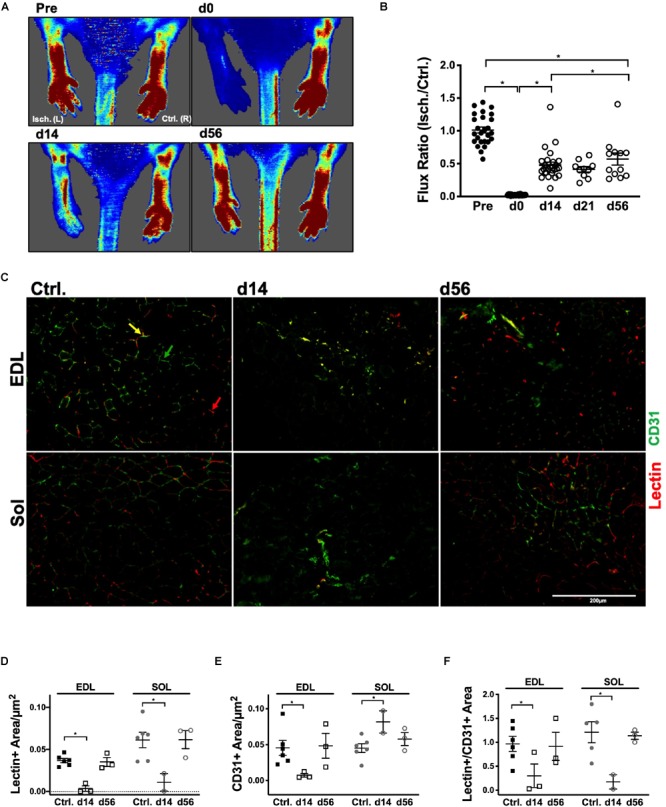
Restoration of peripheral blood flow and muscle capillary perfusion. (A) Representative laser Doppler perfusion images (LDPI) of the ischemic left [Isch. (L)] and control right [Ctrl. (R)] plantar paws in the prone position at baseline, d0, d14, and d56. (B) Quantification of flux measurements at baseline, d0, d14, d21, and d56 represented as a ratio of the left (Isch.) over right (Ctrl.) limbs. (C) Representative 20× immunofluorescent images of systemically DyLight 594 conjugated GS-IB_4_ lectin, which indicates vessels which were actively perfused at the time of sacrifice (red), and cluster of differentiation 31 (CD31^+^, PECAM-1; green), which indicates total endothelial cells, in transverse sections of the control, d14 and d56 Sol and EDL. Red arrows indicate lectin^+^ vessels; green arrows indicate CD31^+^ vessels and yellow arrows indicate CD31^+^/lectin^+^ vessels. (D) Average lectin positive area per μm^2^ in control, d14 and d56 EDL and Sol. (E) Average cluster of differentiation 31 (CD31), area per μm^2^ in control, d14 and d56 EDL and Sol. (F) Ratio of lectin positive area:CD31 positive area in control, d14 and d56 EDL and Sol. Error Bars indicate mean ± SEM. ^∗^*P* < 0.05.
